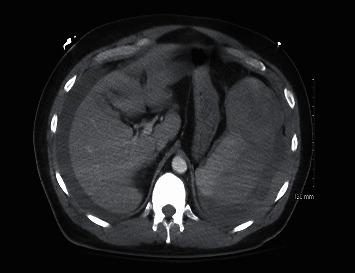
Initial computed tomography scan (CT) of the abdomen showing perihepatic and perisplenic fluid, and the lateral margin of the spleen indistinct with low density areas, concerning for splenic injury or rupture.
